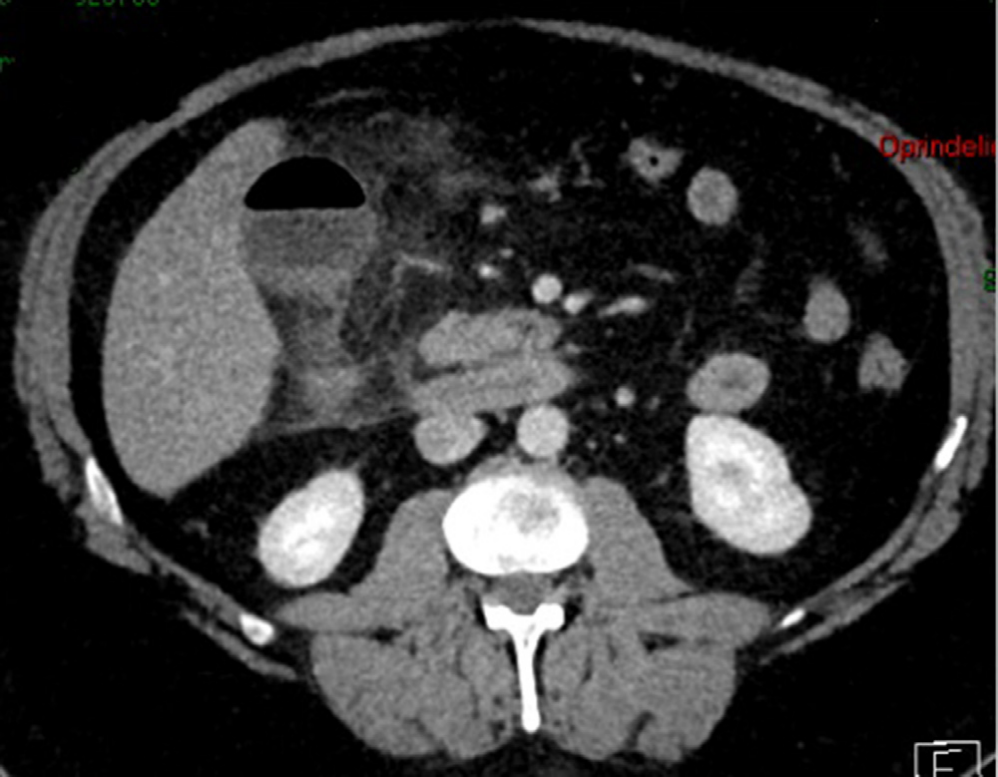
A contrast-enhanced CT scan axial view showing acute emphysematous cholecystitis. Air-fluid level in the lumen of gallbladder with wall thickening, increased enhancement in the gallbladder wall, inflammatory stranding of surrounding fat planes.
